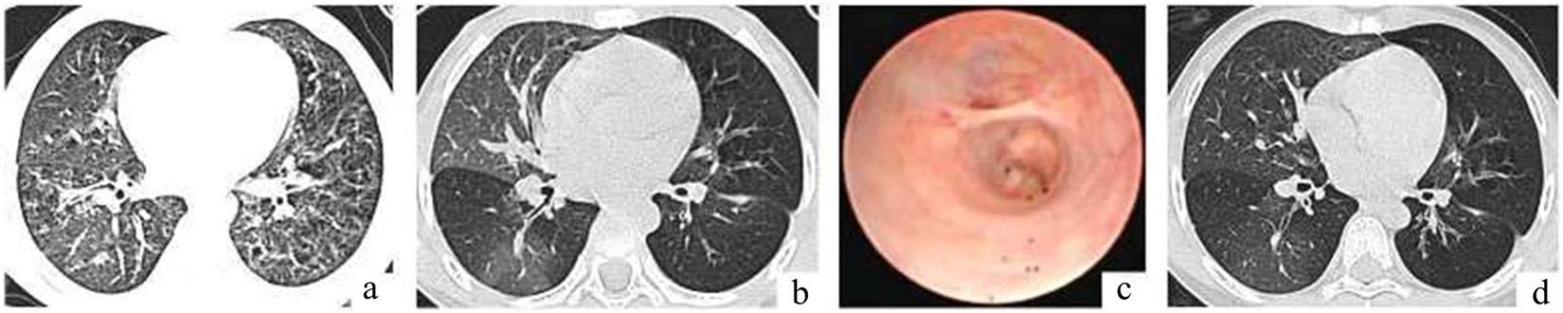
Representative HRCT and Bronchoscopy findings of a patient with moderate disease. (a) HRCT showing inflammatory bronchiolitis. (b) HRCT showing mosaic patterns, bronchiectasis and left lung hyperlucency. (c) Bronchoscopy showing a partially occluded lumen in segmental bronchi. (d) HRCT showing BO after 4 years of treatment.
